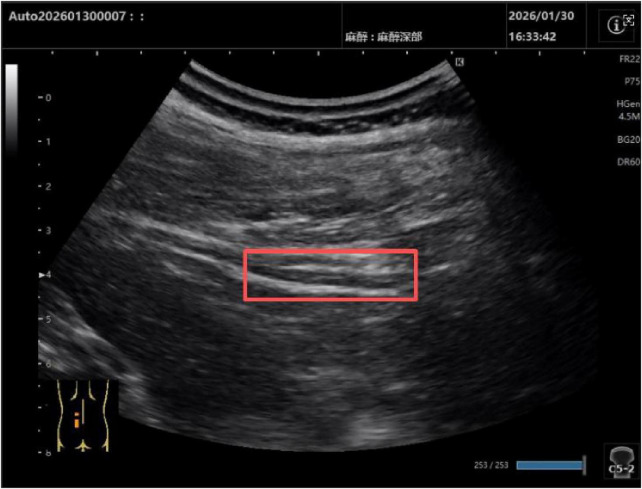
Muscle fiber changes on musculoskeletal ultrasound (SONIMAGE MXI PRO).
